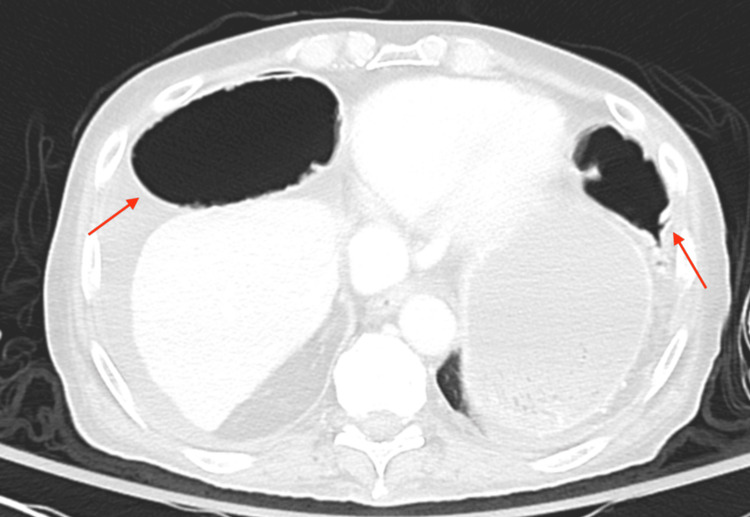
Axial upper cut of CT abdomen and pelvis with IV contrast showing intraperitoneal air on the left and right of heart consistent with perforated viscus (red arrows). Trace ascites are noted CT: computed tomography
